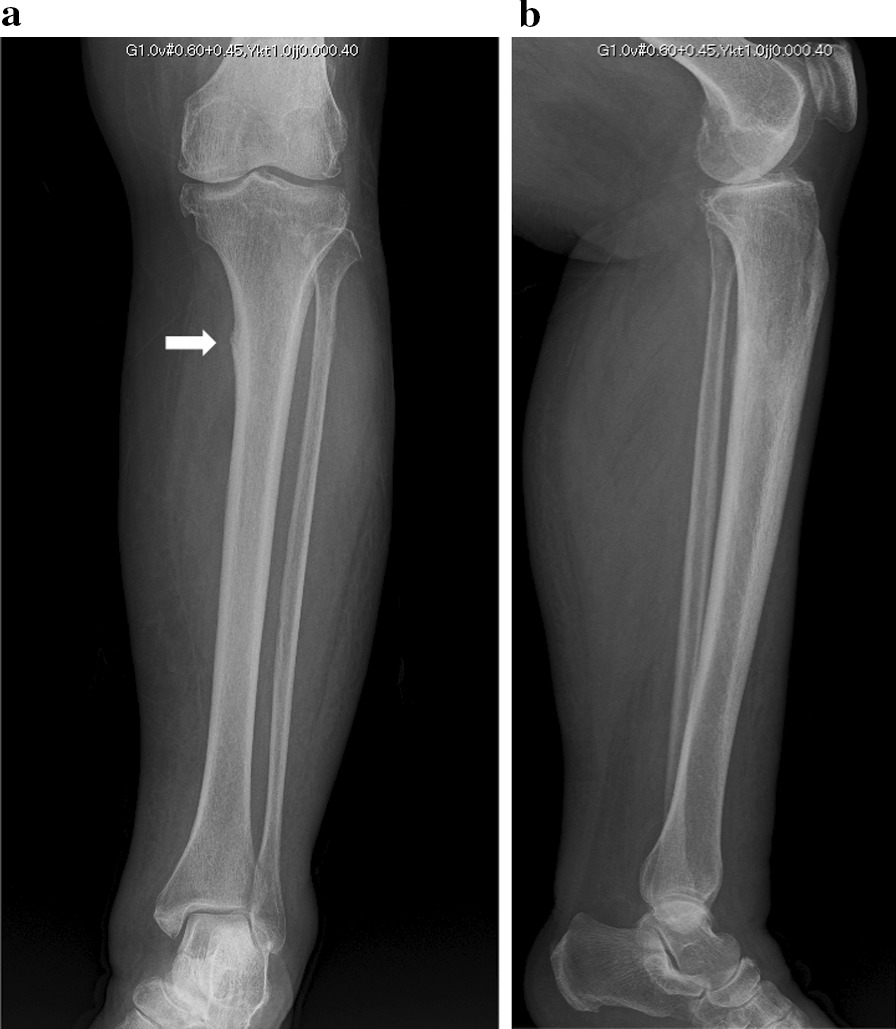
X-ray images at the initial visit for her shin pain. The “beaking sign” (white arrow) occurred due to focal endosteal thickening of the medial cortex in the proximal third of the tibia
